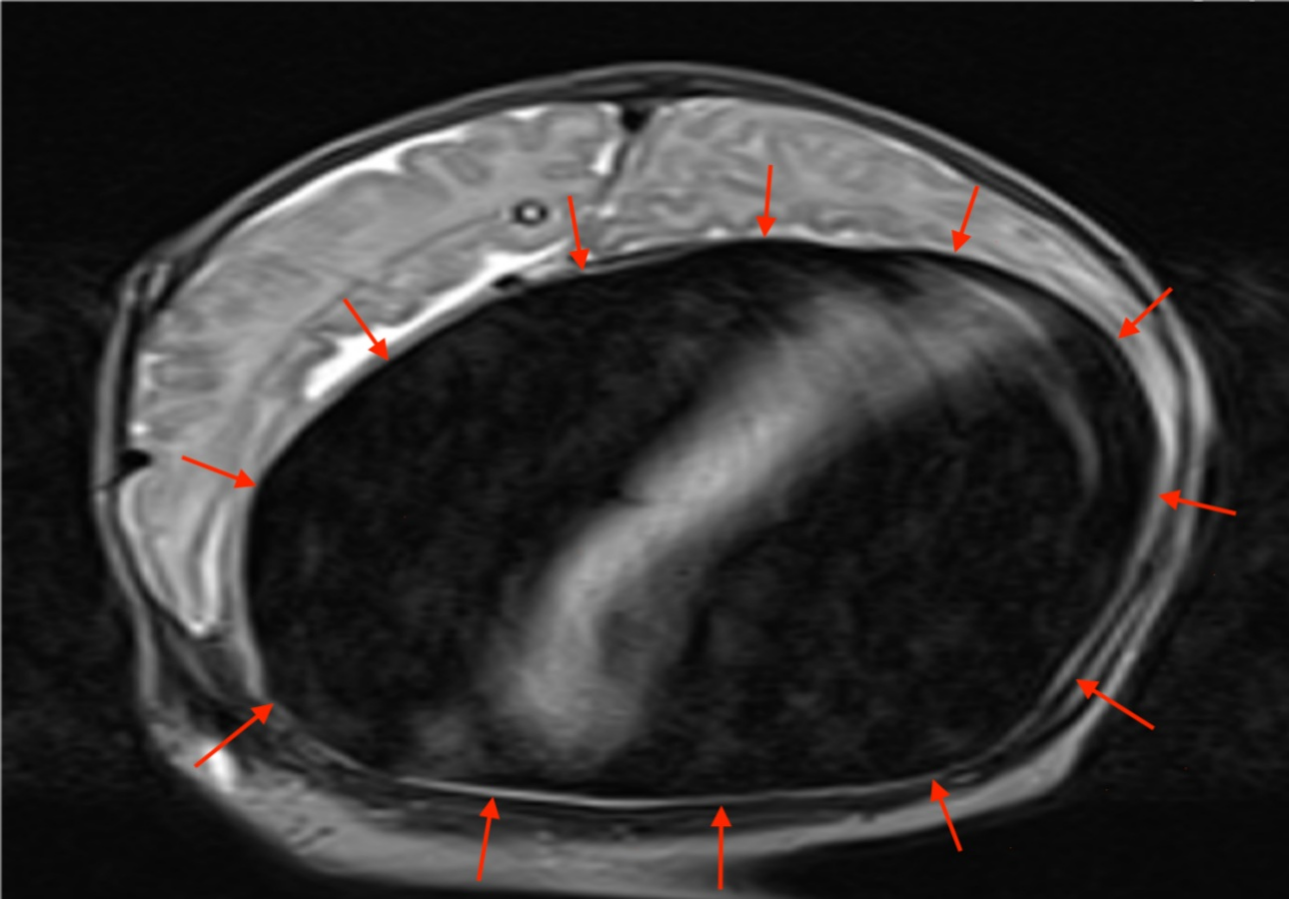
Coronal T2-weighted MRI of the patient above noting the large VGAM (red arrows) MRI: magnetic resonance imaging; VGAM: vein of Galen aneurysmal malformation.
